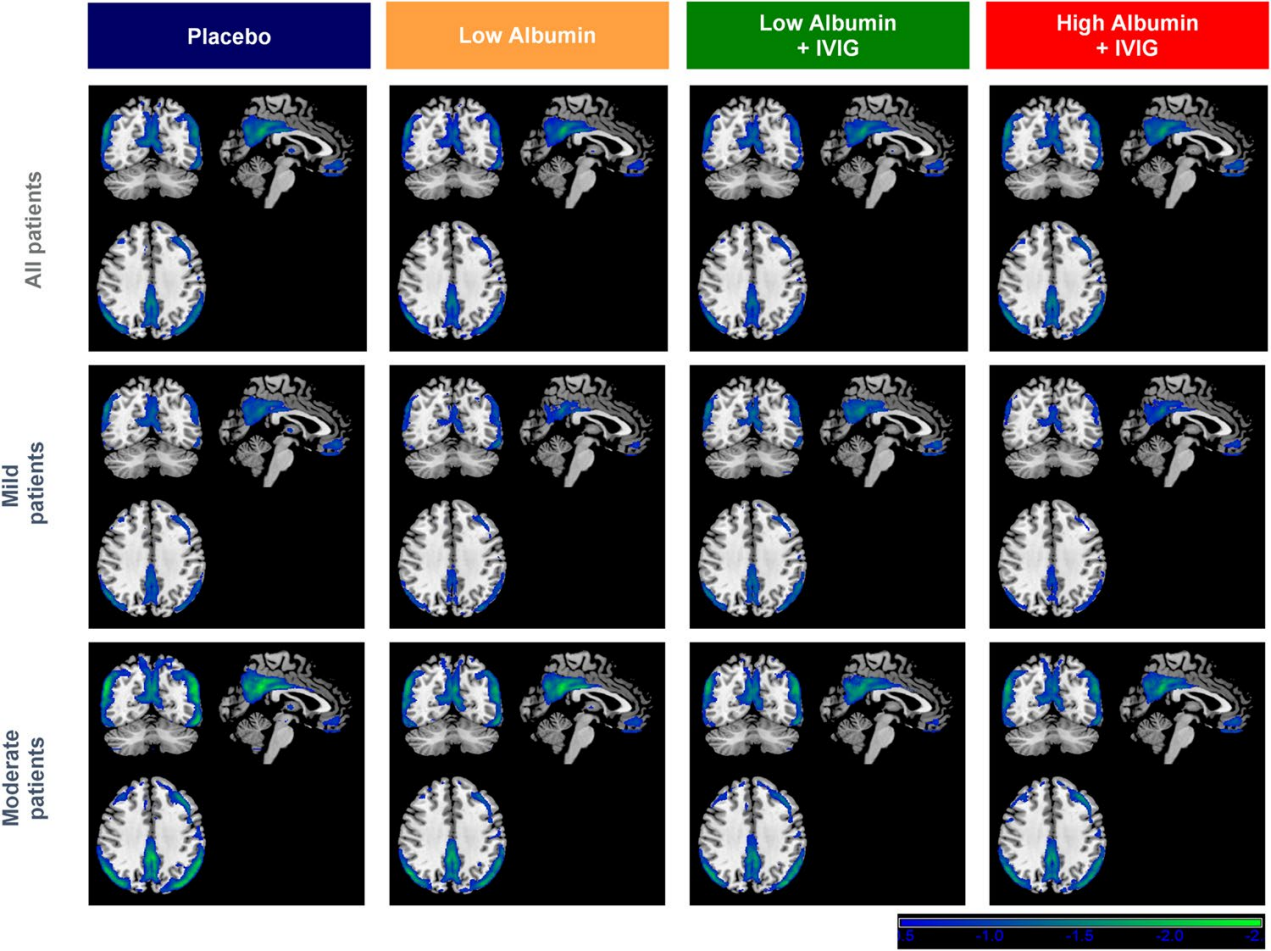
Defect pattern in ^18^F-flurodeoxyglucose positron emission tomography (^18^FDG-PET) analysis at baseline in Alzheimer’s disease patients treated with plasma exchange with low- or high-dose albumin with or without intravenous immunoglobulin (IVIG). Axial view
